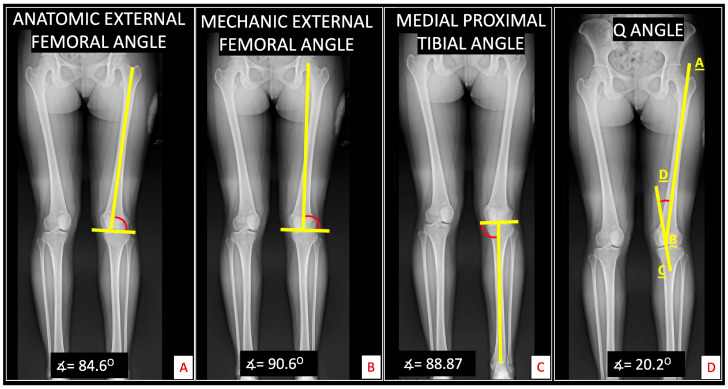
Radiographic evaluation in the panoramic projection. Panoramic lower extremity X-Ray was used to measure (A) the anatomic external femoral angle, with tolerable values between 79° and 93°; (B) the mechanic external femoral angle, with tolerable values between 85° and 90°; (C) the medial proximal tibial angle, with tolerable values between 85° and 90°; and (D) Q angle, with tolerable values between 8–15° for women and 8–10° for men. Obtained by the angle formed by the intersection of lines AB (Anterior superior iliac spine- Center of patella) and CD (Tibial Tuberosity-Line passing the center of patella).
